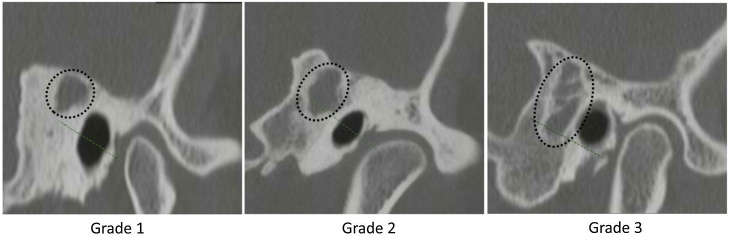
The Computed Tomography (CT) images of temporal bone in the coronal plane revealed the position and size of cholesteatoma marked by the dotted line circles, which were scored as Grade 1, 2 and 3 in this study.
